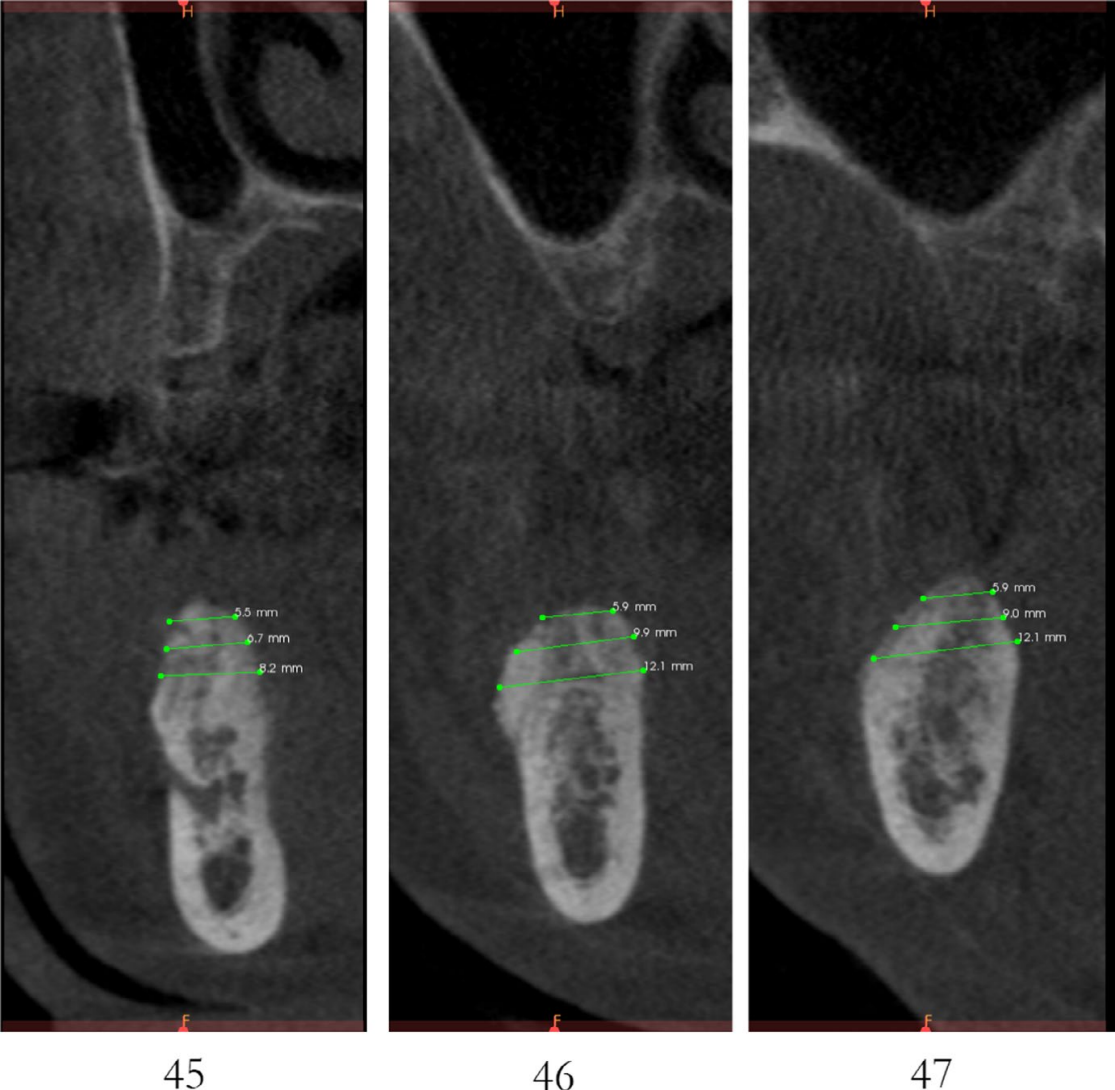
CBCT scanning 6 mo after the bone operation
